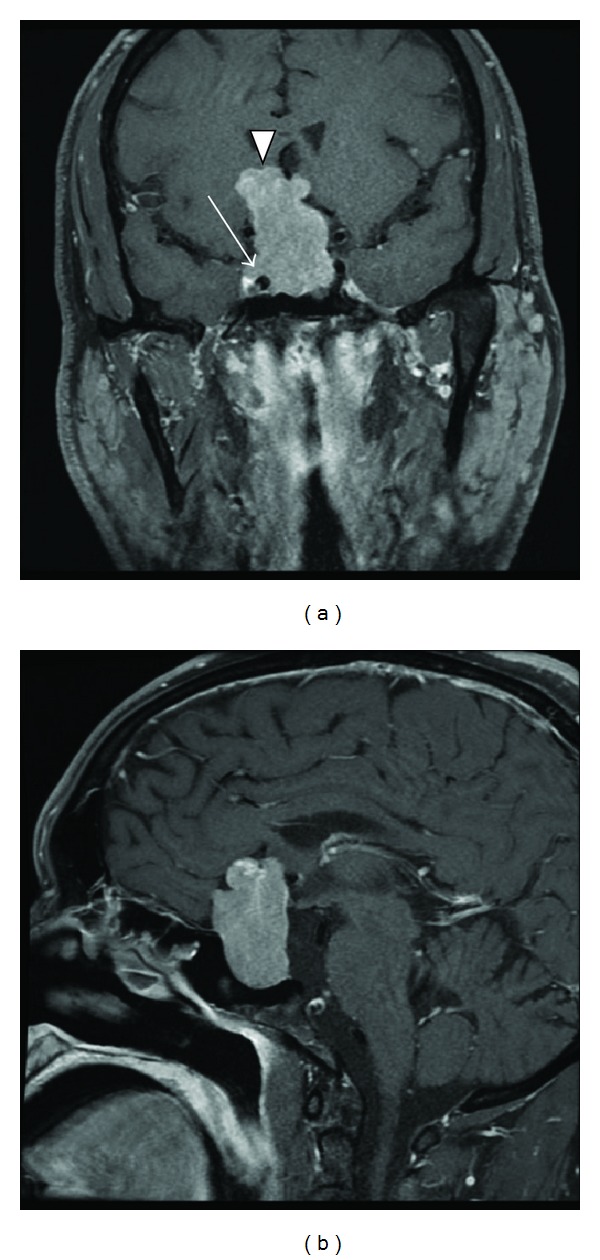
MRI of the sella. T1-weighted, postgadolinium coronal (a) and sagittal (b) images show a hyperdense lesion that expands the sella and extends intracranially (arrow head) through the suprasellar space to compress the optic nerves and chiasm. In the coronal view, one can see the tumor wrapping above the right carotid artery in the cavernous sinus (arrow).
